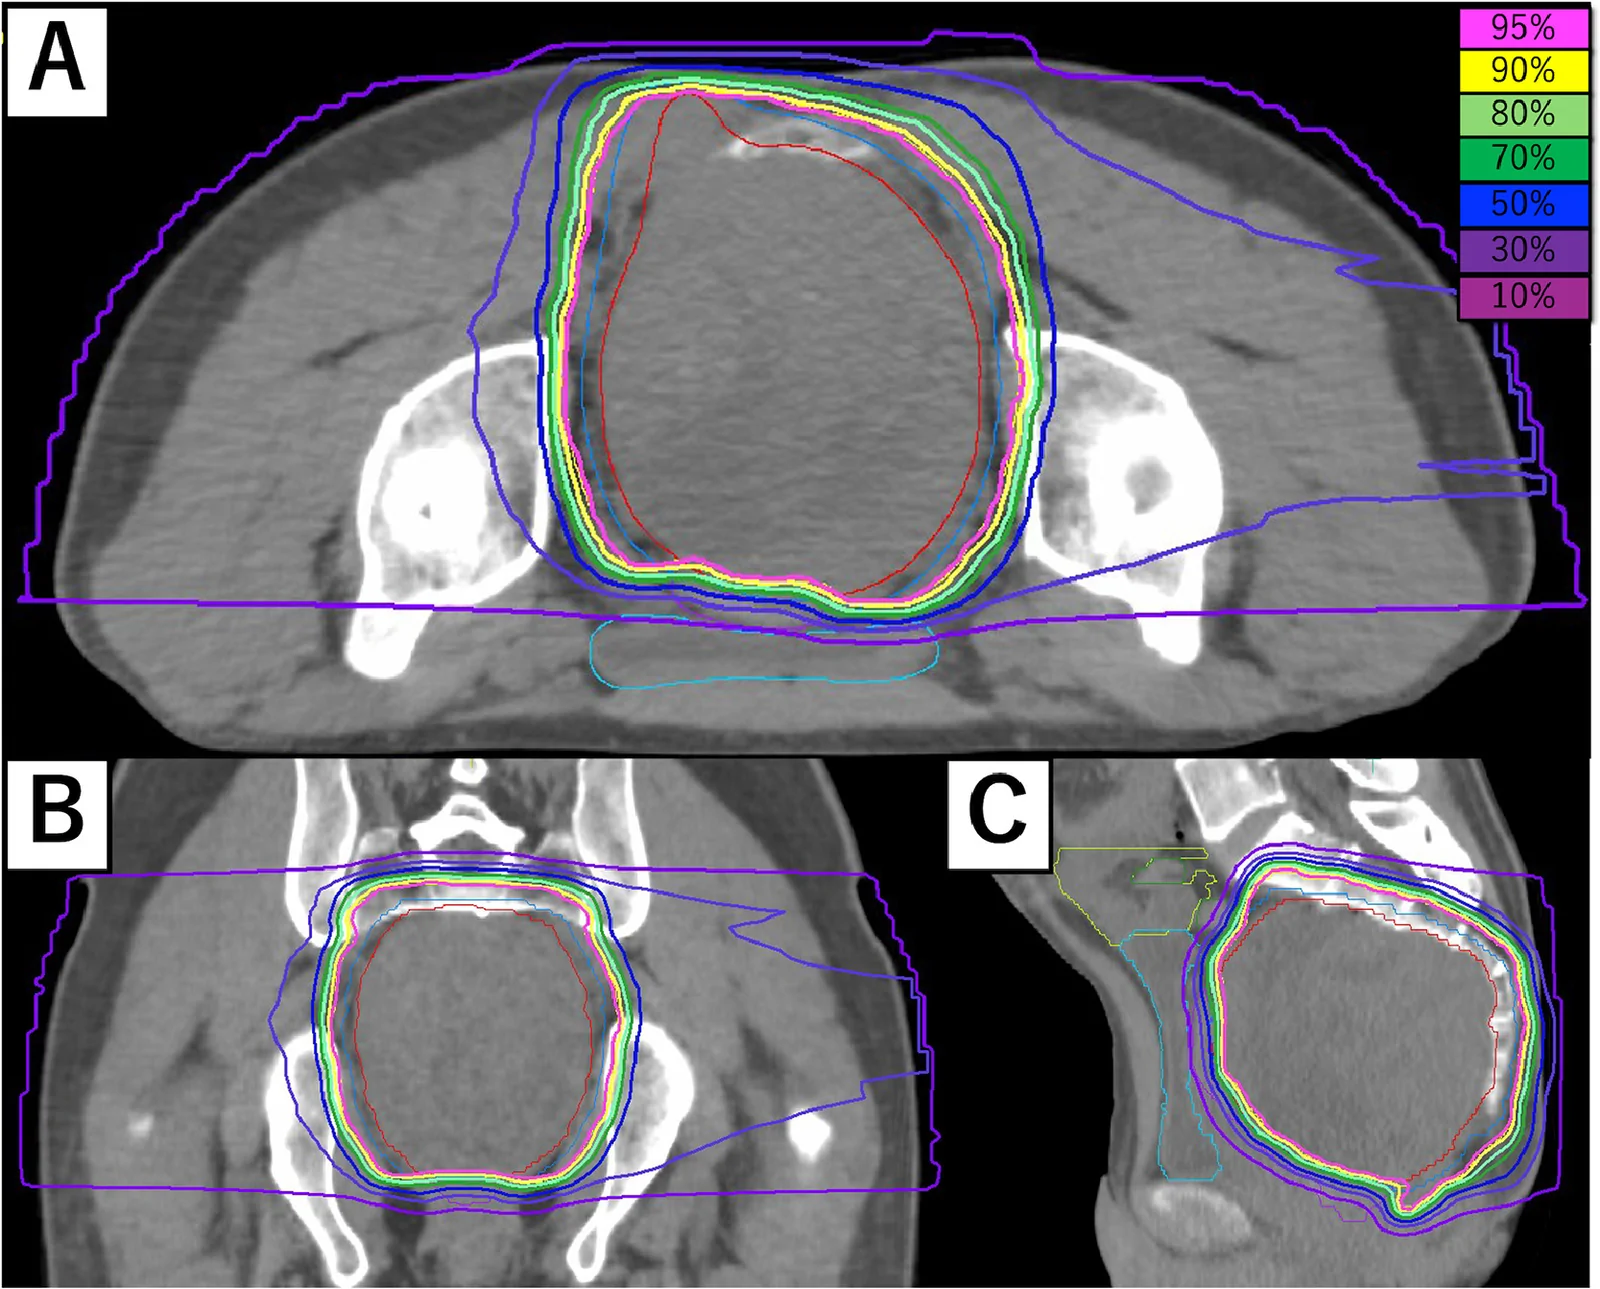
A 38-year-old male patient with a solitary fibrous tumor in the presacral region treated with carbon-ion radiation therapy (70.4 Gy in 16 fractions). Dose distribution on (A) axial, (B) coronal, and (C) sagittal computed tomography images. The area outlined in red indicates the gross tumor volume. The colored areas represent the 95% (pink), 90% (yellow), 80% (light green), 70% (green), 50% (blue), 30% (purple), and 10% (light purple) isodose curves.
